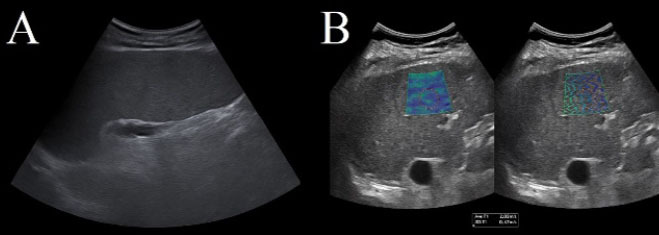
In A, Conventional B-mode liver ultrasound showing
